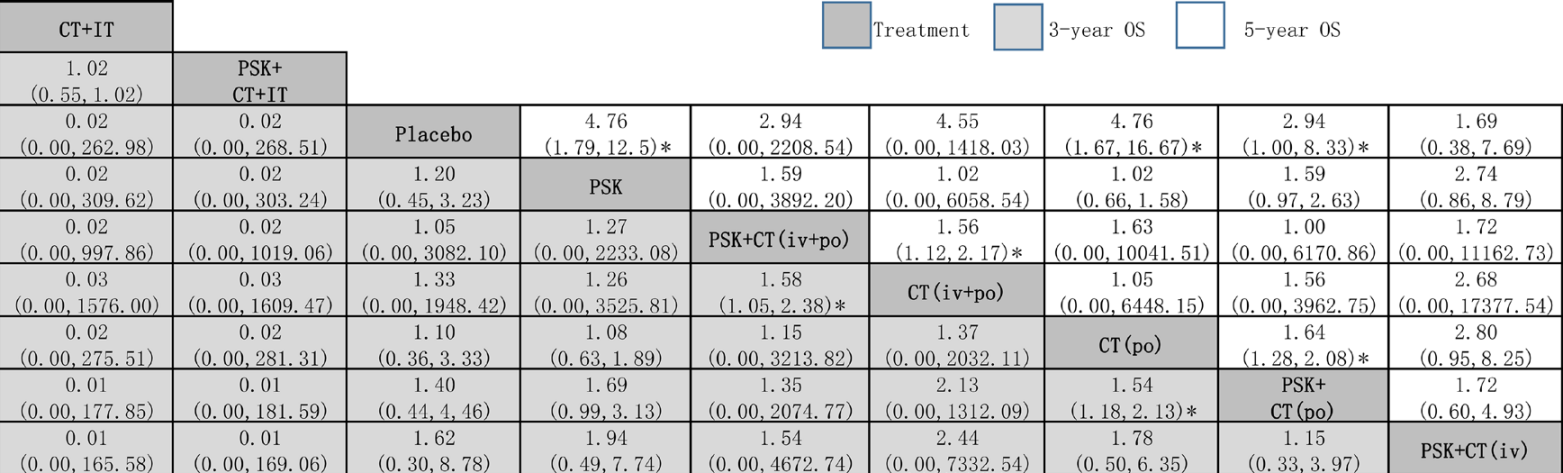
Network meta-analysis of 3-year OS and 5-year OS The treatments are reported in order of survival rate ranking according to SUCRA of 3-year OS. Comparisons should be read from left to right. The estimate is located at the intersection of the column-defining the treatment and the row-defining the treatment. For OS, a OR value above 1 favors the PSK arm. *Result with significant difference. Abbreviation: CT, chemotherapy; IT, immunochemotherapy; OS, overall survival; PSK, Polysaccharide K.
